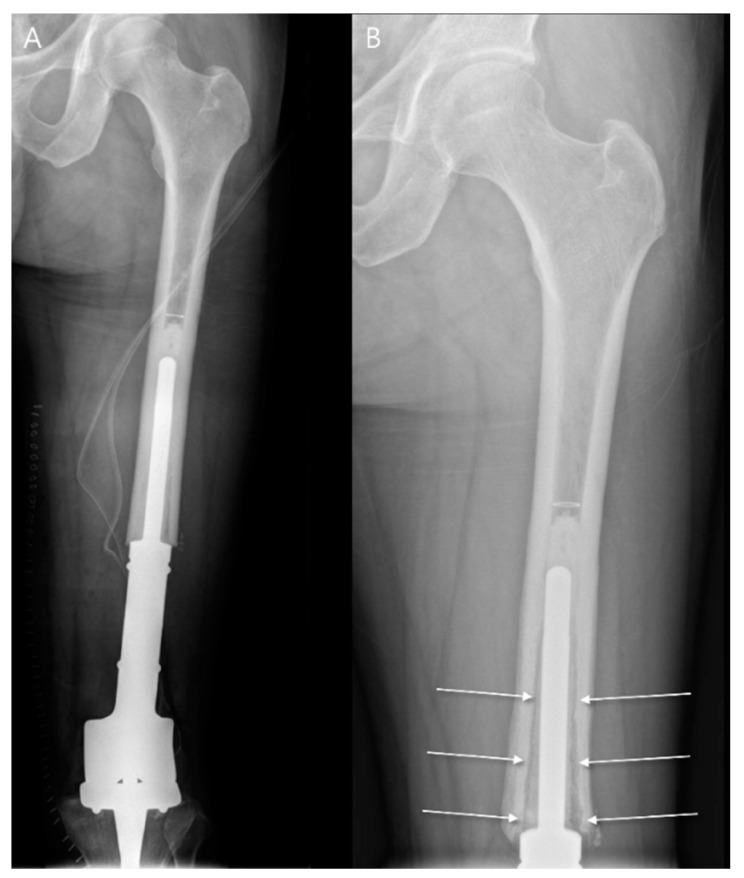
Aseptic loosening following limb salvage surgeries for low-grade central osteosarcoma. (A) Immediate postoperative plain radiograph is shown. (B) One-year follow-up plain radiograph shows a diffuse radiolucent zone at the metal–bone interface, measuring more than 1 mm (arrows), suggesting the implant is “probably loose”.
